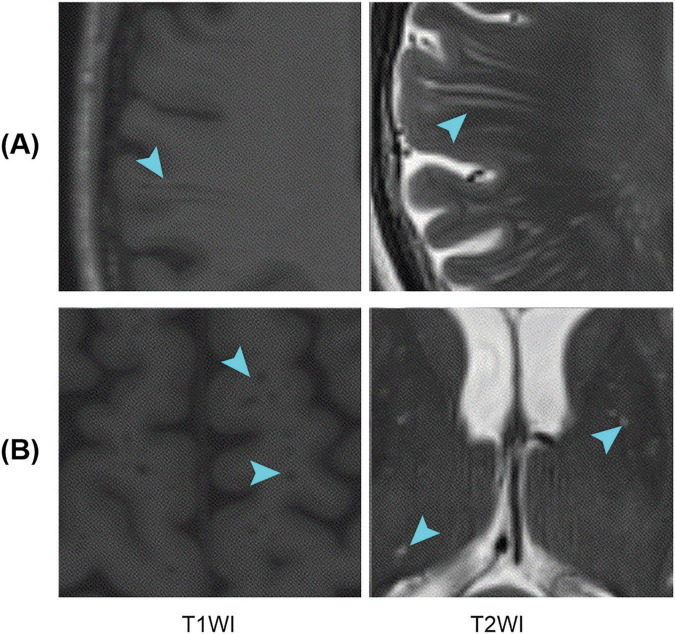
Examples of PVSs on magnetic resonance images. T1WI represents T1-weighted images, and T2WI represents T2-weighted images; (A,B) Indicate that the PVSs appear as lines and dots, respectively. PVSs, perivascular spaces.
